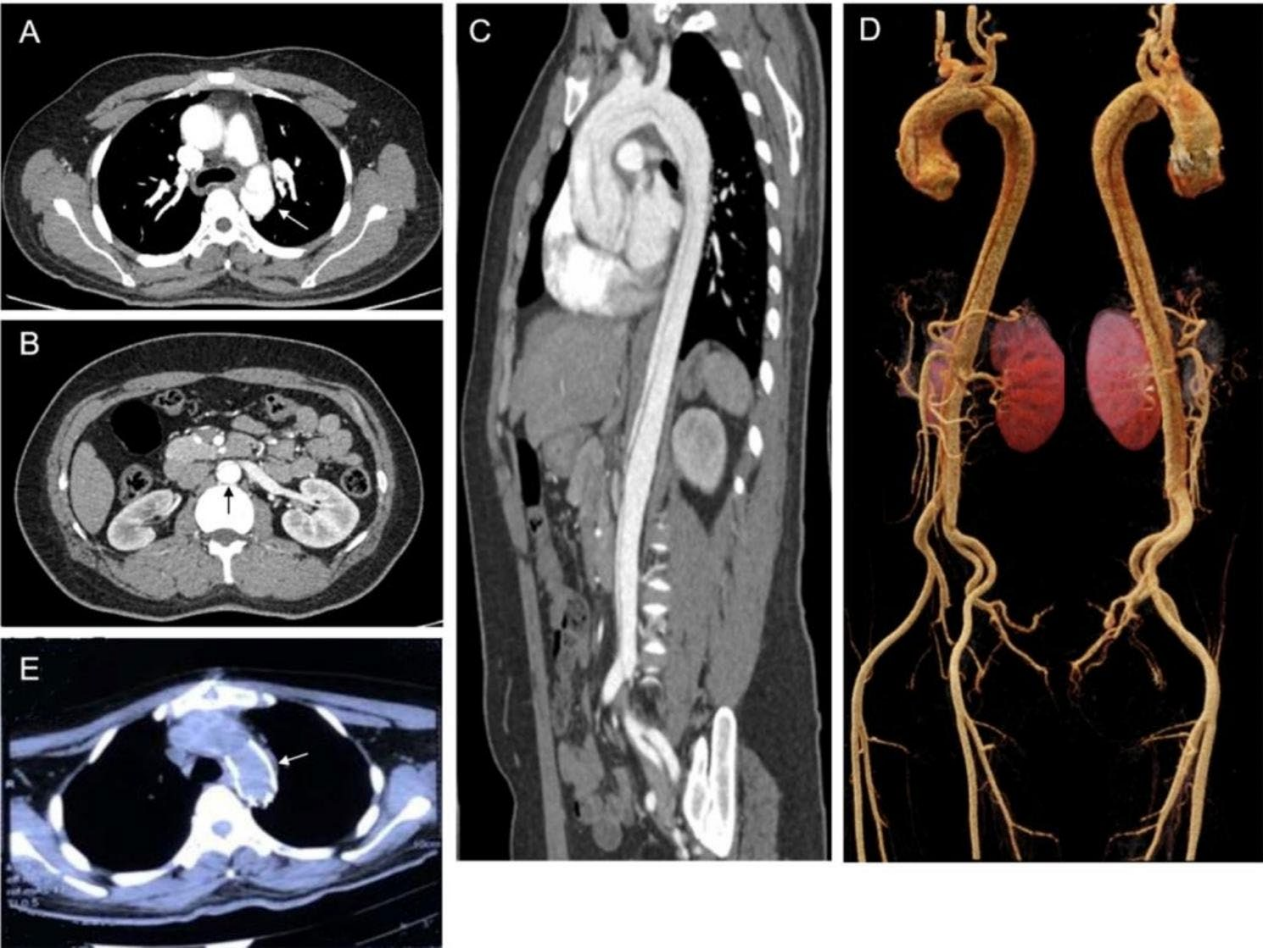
Images of aortic dissection in the patient before and after the operation. (A) Contrast-enhanced CT image of aortic dissection in the aortic arch. (B) Contrast-enhanced CT image of aortic dissection in the abdominal aorta. (C) CT angiography of aortic dissection on sagittal reconstruction. (D) Three-dimensional reconstruction of the aorta demonstrating aortic dissection (Stanford type A). (E) CT image of the aortic arch after the operation. (The arrow represents the position of the lesion in the aorta.)
